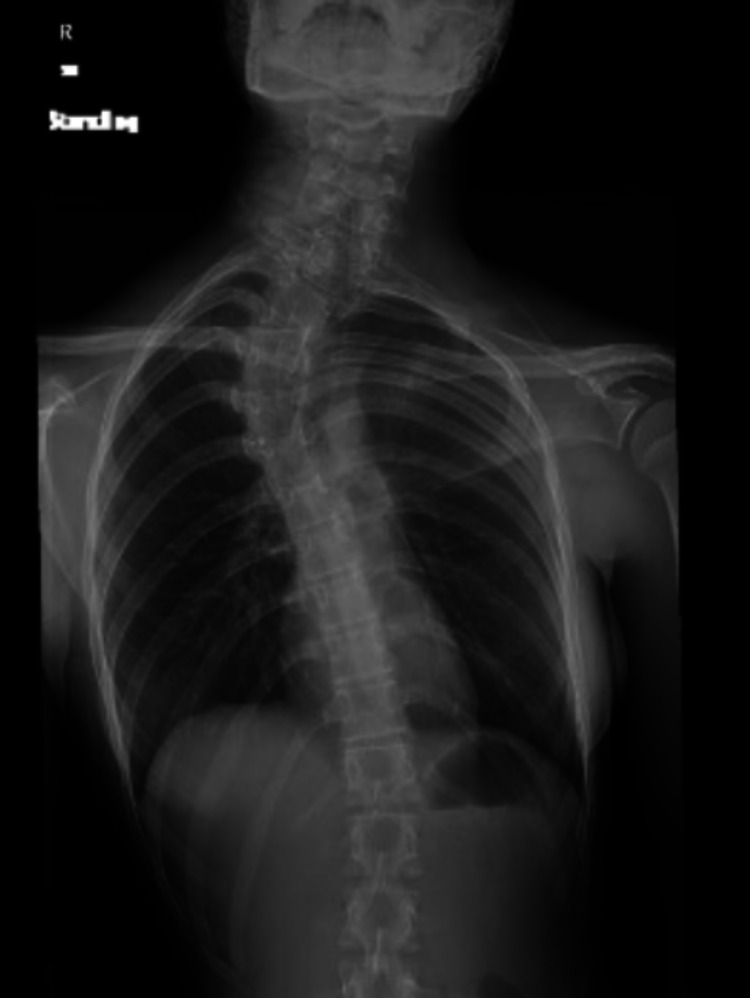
Plain AP radiograph of the patient showing congenital scoliosis and elevated left scapula AP: anteroposterior
